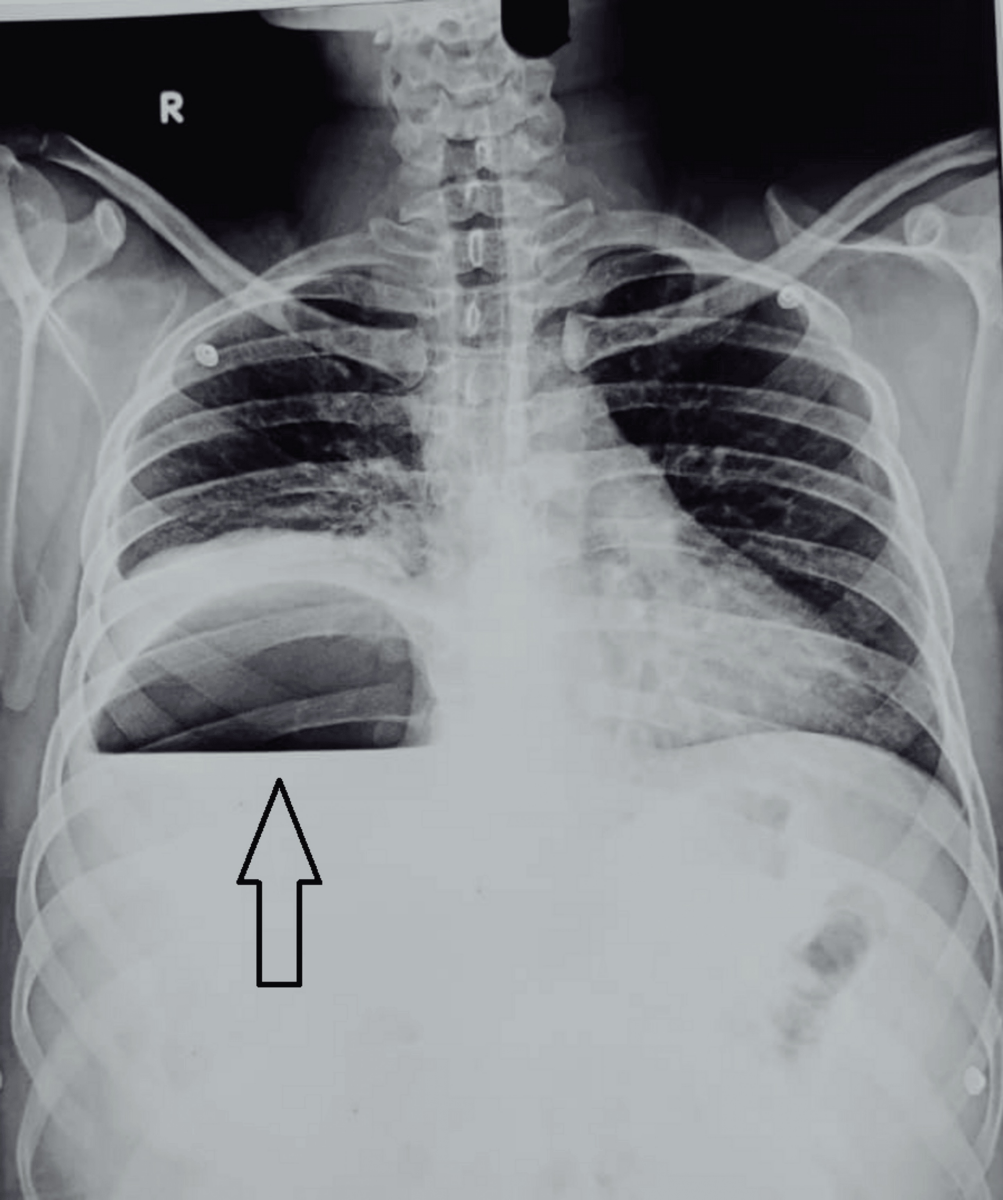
Abdominal X-ray of patient 4 showing gas under the right dome of the diaphragm (arrow)
